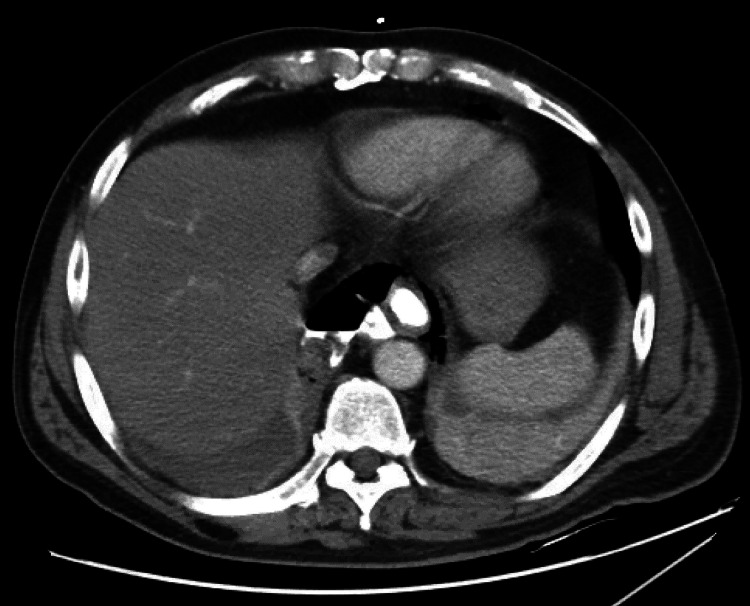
Continuation of CT in Figure 1, with better demonstration of extravasated oral contrast and air within the mediastinum
